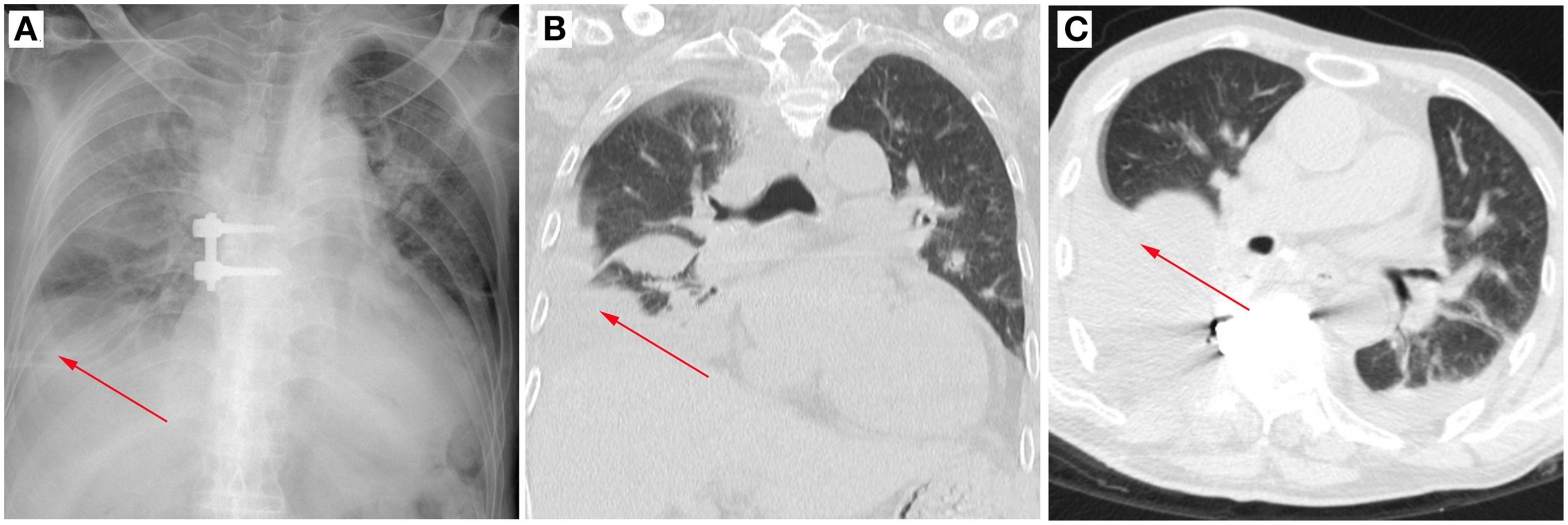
A typical case of post-operative pleural effusion after spinal tuberculosis. The arrow marks the location of the pleural effusion. (A) Post-operative X-ray image in the posterior–anterior position. (B) Post-operative CT image in the coronal position. (C) Post-operative CT image in the cross-section.
